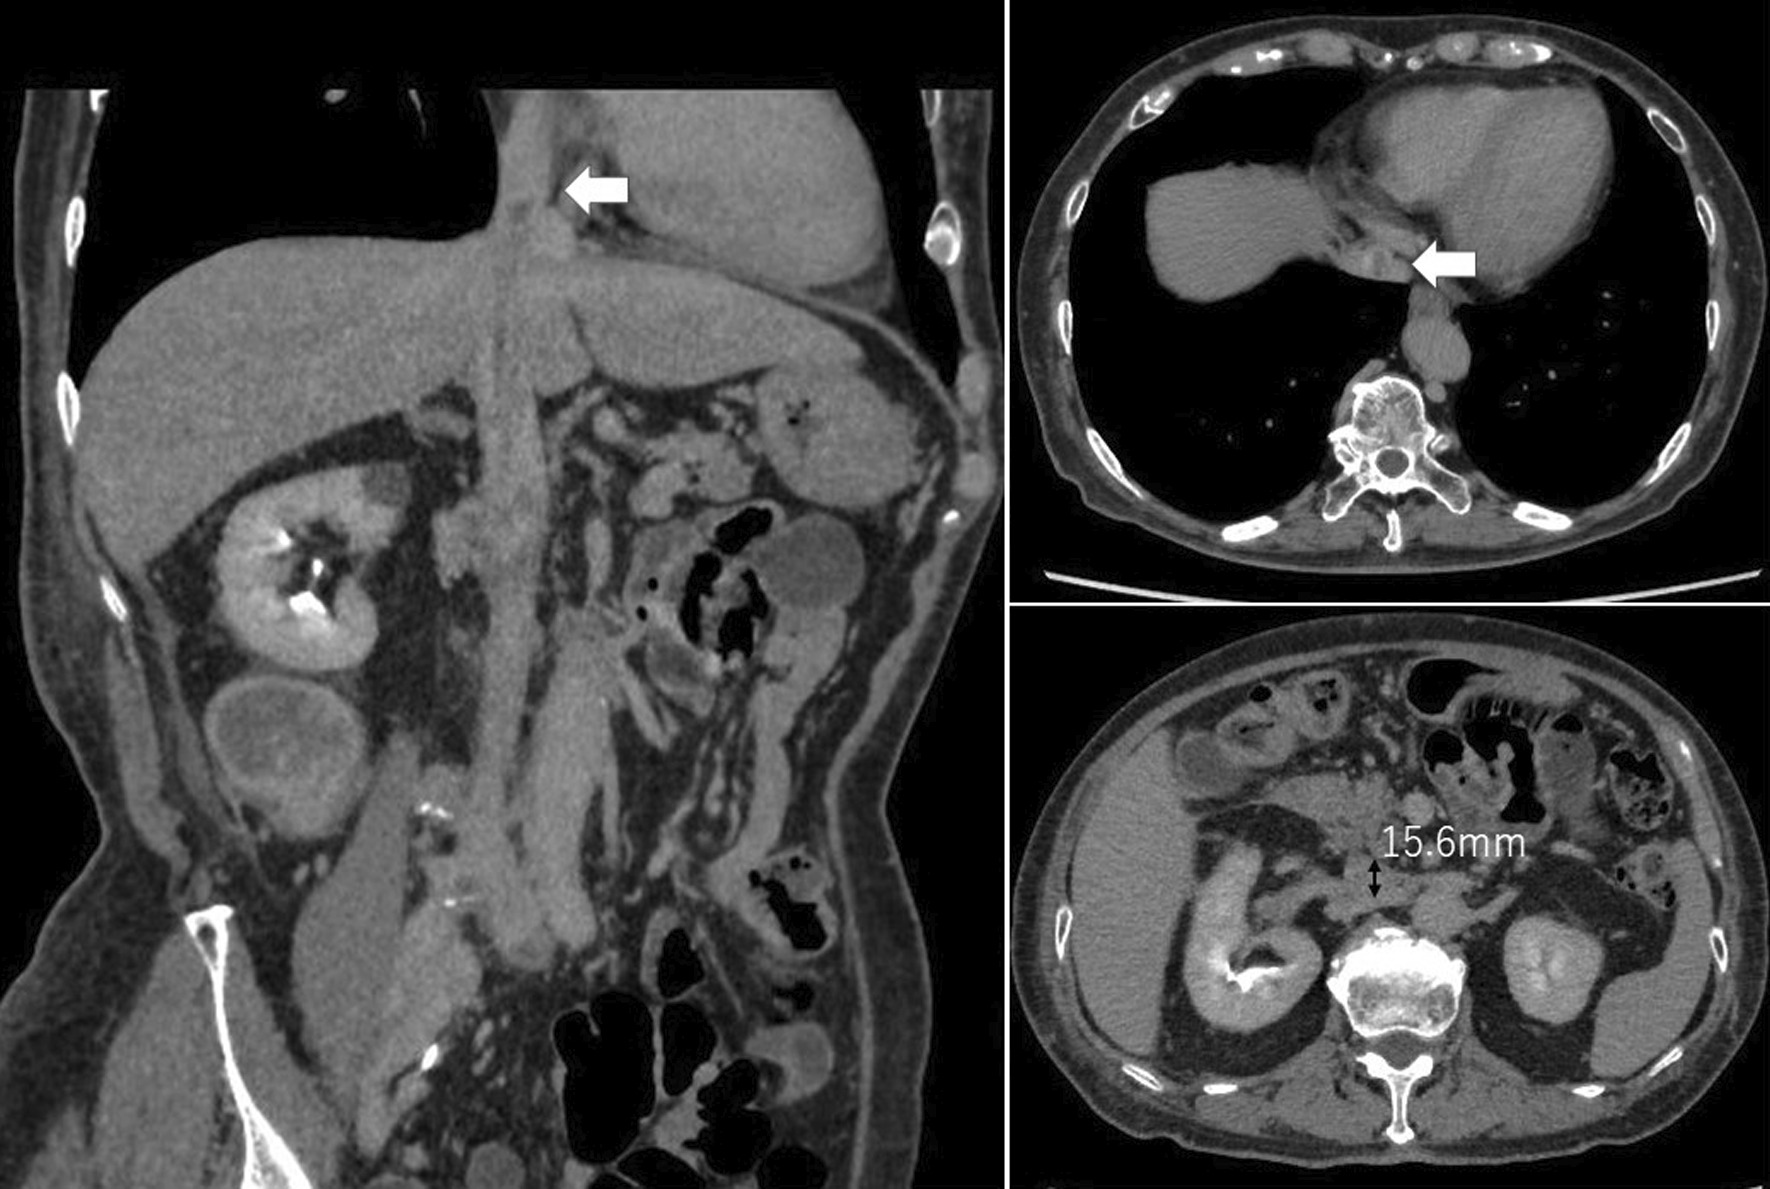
Characteristics of the preoperative tumor thrombus on enhanced CT. The white arrows indicate the tip of the tumor thrombus. The black arrows indicate the diameter of the IVC at the renal vein ostium (15.6 mm)
